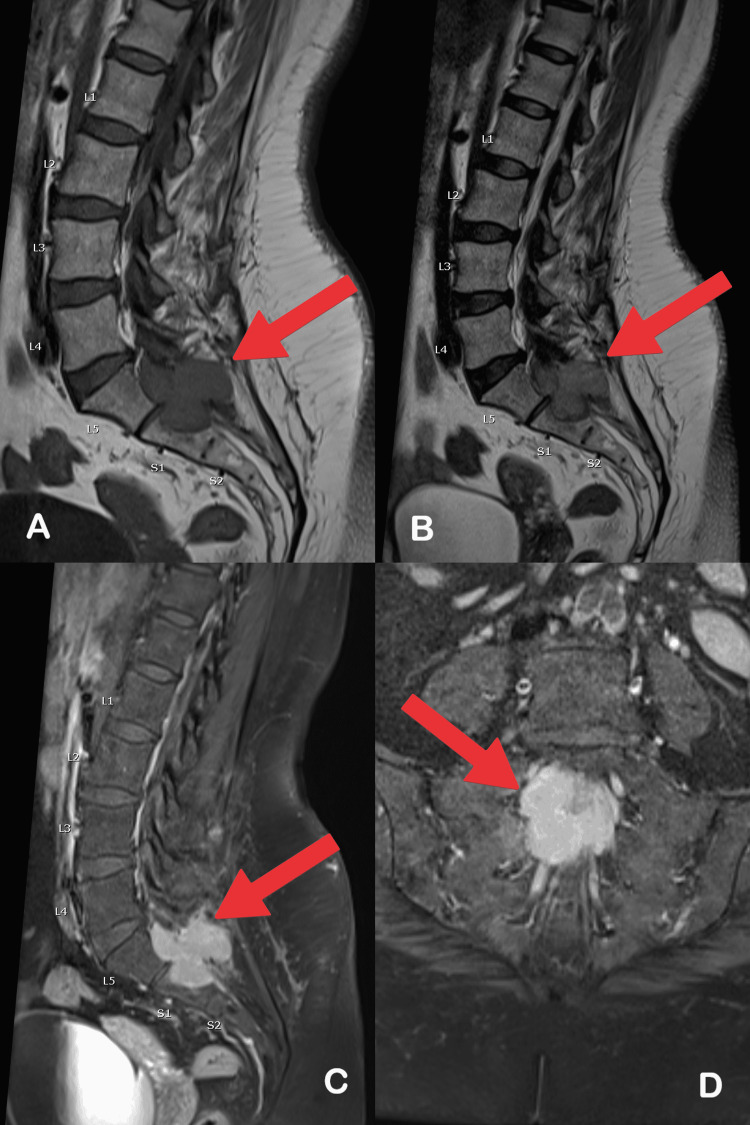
(A) Sagittal T2, (B) Sagittal T1, (C) Sagittal post-contrast, and (D) Axial post-contrast MRI images of the lumbosacral spine demonstrating large, lobulated, expansile, intradural, T2 hyperintense, T1 isointense, enhancing soft-tissue mass located at the level of L5-S1. Adjacent vertebrae are intact. There is severe bone remodelling at the posterior aspect of S1 and S2 vertebrae along with posterior soft-tissue invasion.
